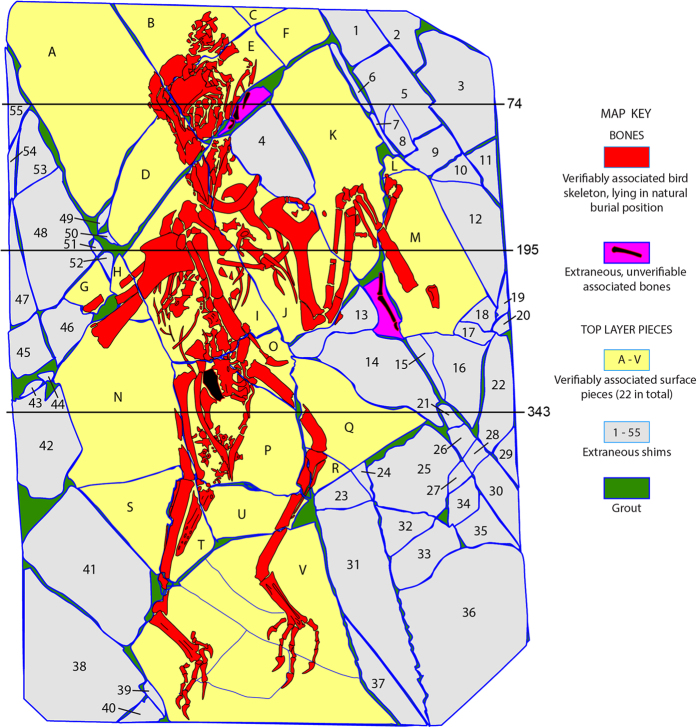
Map of the *Confuciusornis* amalgamation derived from high-resolution X-ray CT data (Data Citation 1). The skeleton is in red and its associated shale is yellow. Extraneous bone-bearing pieces are magenta, construction shims are gray, and grout is green. The numbered lines indicate the positions of slice planes for original CT data labeled in Figs 4 and 6.
